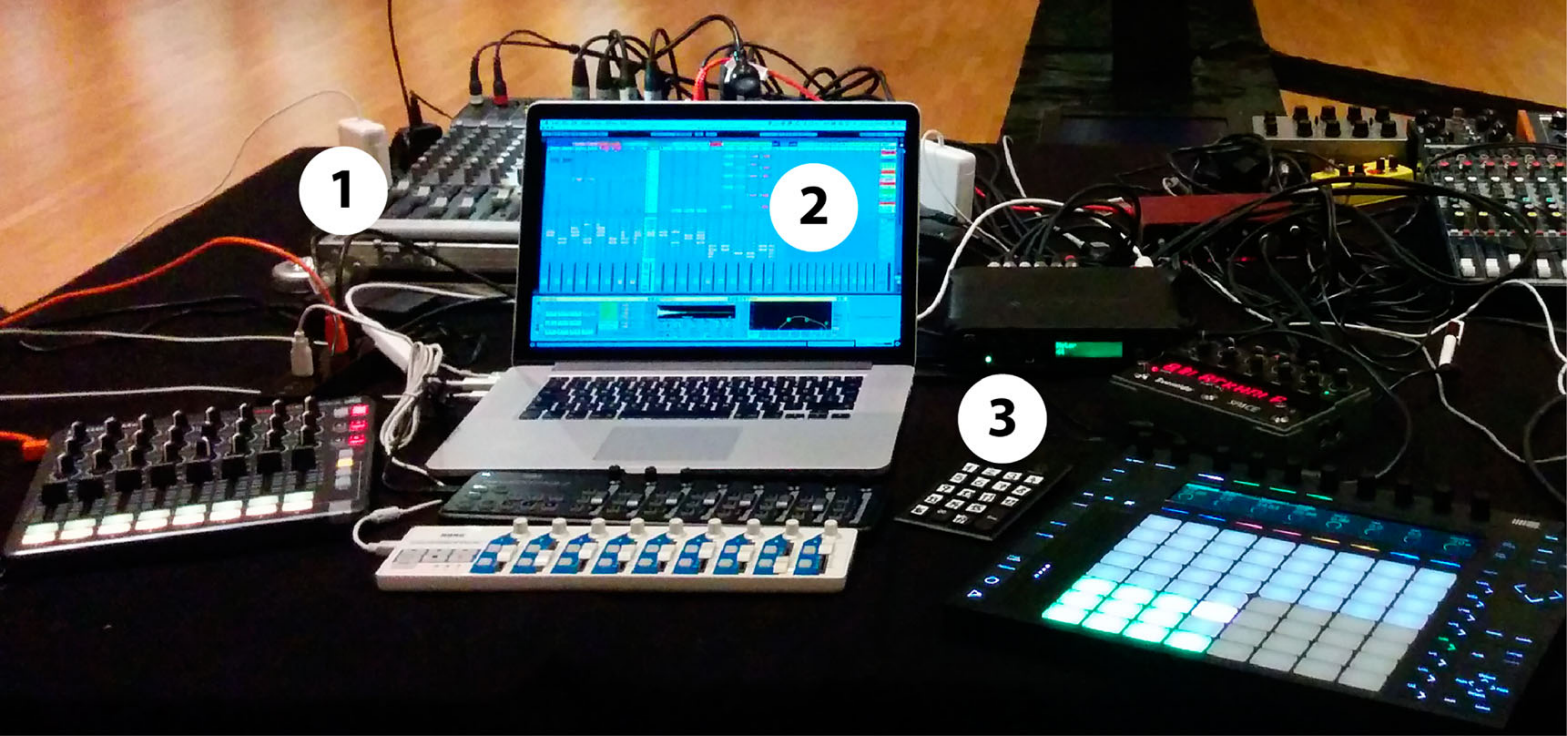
The DJ setup at the centre stage with a (1) mixer, (2) laptop, and (3) keypad to send ultrasound IDs as further described in the text.
